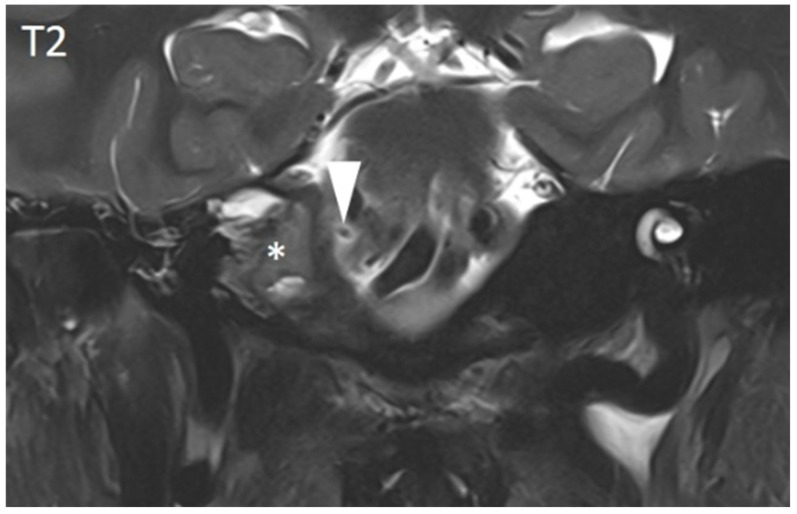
MRI (with contrast agent) of a young patient with clinically present Gradenigo syndrome. The image of an inflammatory pseudotumor in the petrous apex (star) correlates with the computed tomography images. The coronary T2 images allow the direct visualization of the abducens nerve (arrowhead) adjacent to the inflammatory changes.
